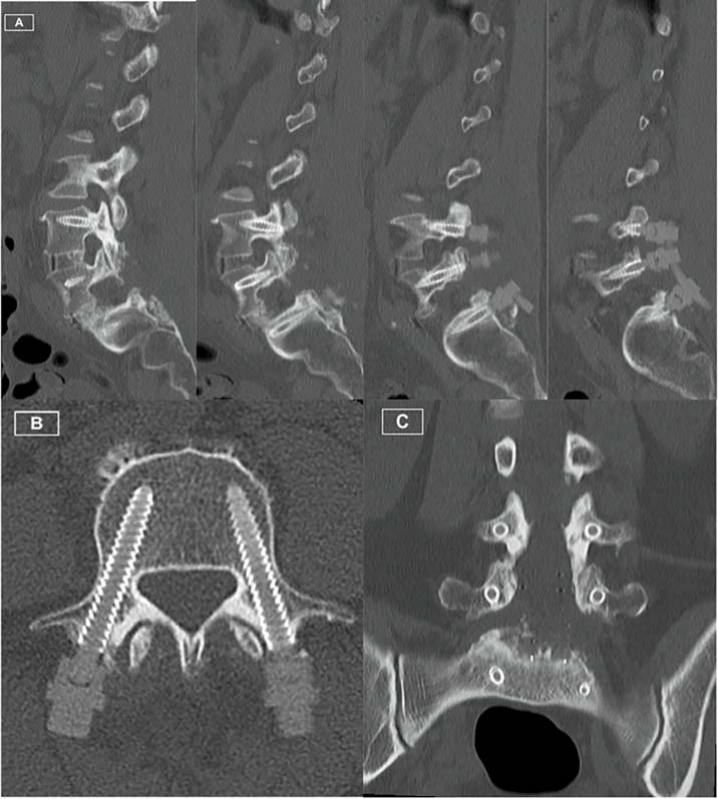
Postoperative CT Lumbar spine. (A) Sequence of sagittal slices demonstrating excellent definition of screw trajectory. (B) Axial slice through L4 pedicles demonstrating definition of screws and distinction from bony cortex. (C) Coronal slice demonstrating screws at all levels with no peri-structural artifact.
